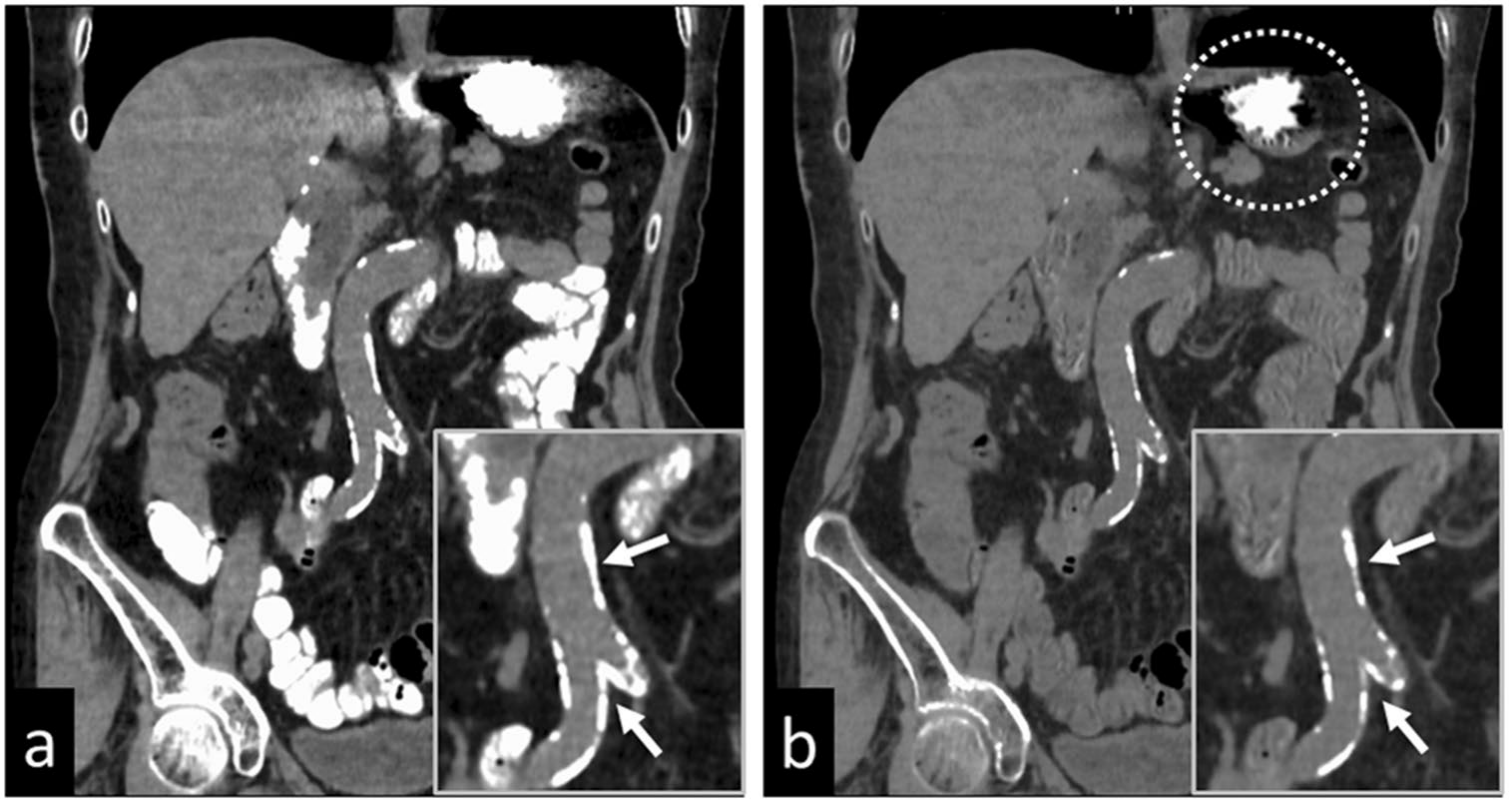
A 77-year-old woman who received gastrografin orally. The virtual monochromatic image at 70 keV (a) and the virtual non-contrast enhanced image (b) were obtained after unenhanced dual-energy CT. On the virtual non-contrast enhanced image (b), iodine in the small intestine is well removed. Iodine removal from the stomach is incomplete (dotted circle), suggesting that the iodine concentration was very high. During the virtual non-contrast reconstruction process, the volume of the calcification on the aortic wall was reduced (arrows)
